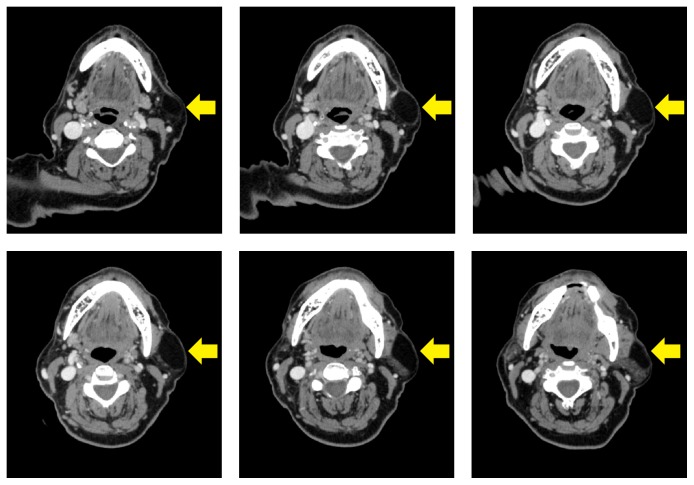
CT scans showed a low density homogeneous capsulated mass with sharp margins in the superficial lobe of the left parotid gland.
